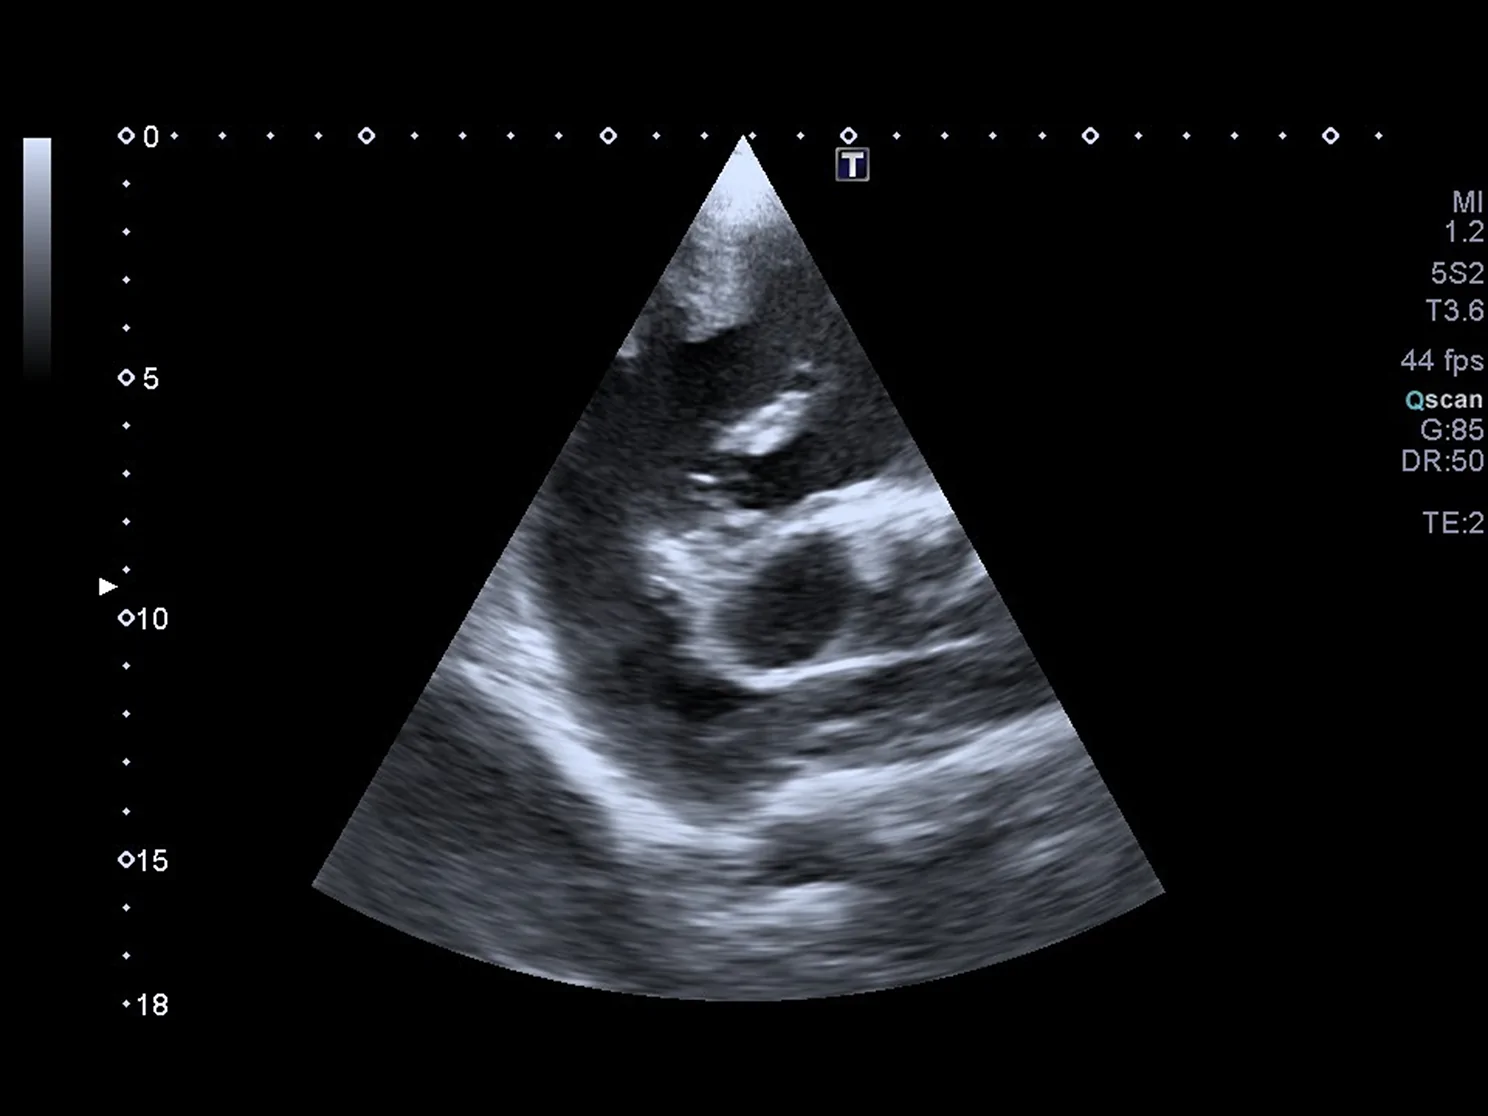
Echocardiographic view in which the aorta appears to arise predominantly from the right ventricle. The image supports suspected DORV but does not, by itself, demonstrate the origin of both great arteries or define the VSD-great-artery relationship. No better archived still frame or cine loop could be retrieved
